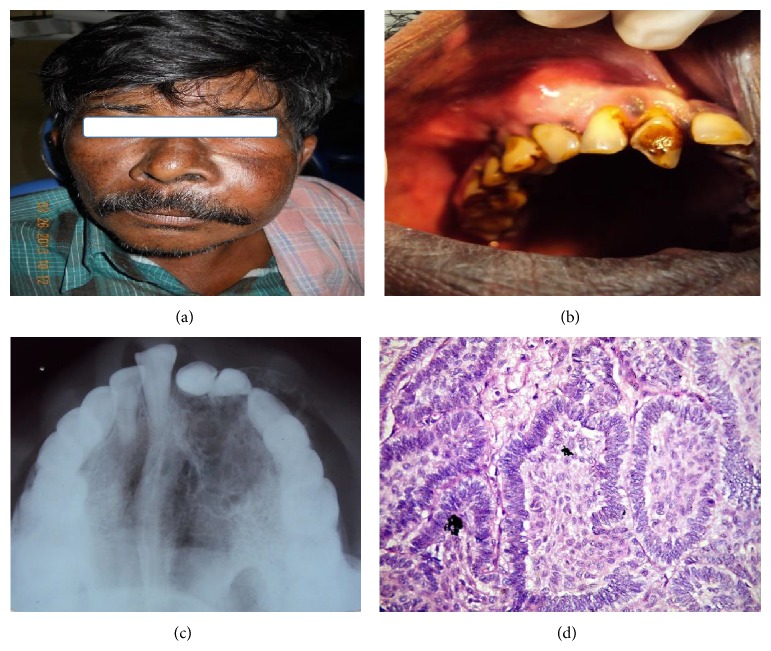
(a-b) Preoperative view. (c) Occlusal radiograph. (d) Follicular type ameloblastoma.
